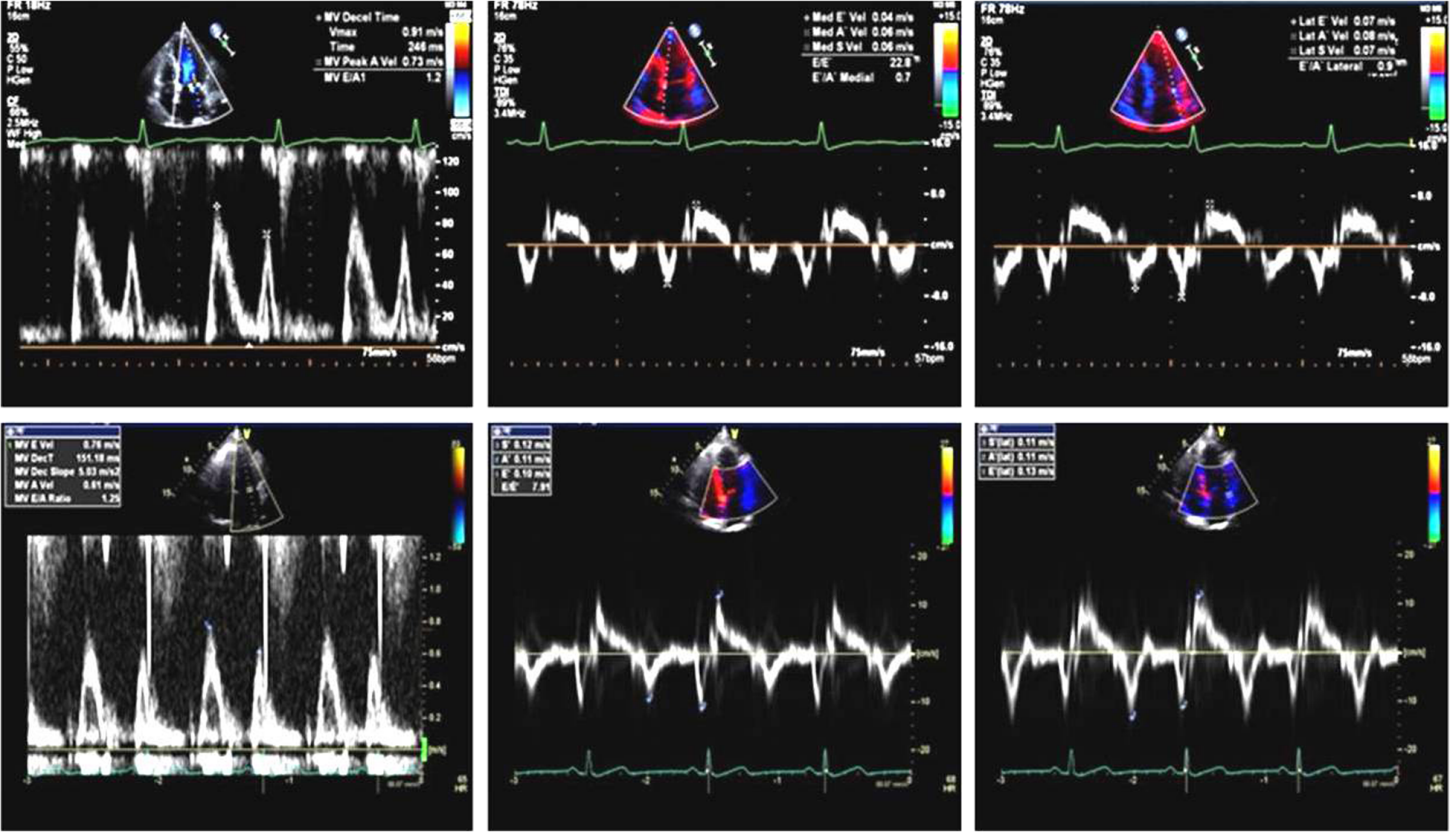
Representative figures of a subject with concordance (upper 3 figures) and discordance pattern (lower 3 figures) of tissue Doppler imaging.
